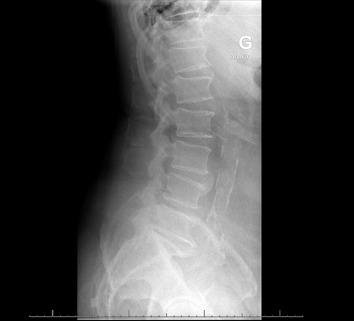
Lumbar X-ray (11.12): no signs of bone destruction.
